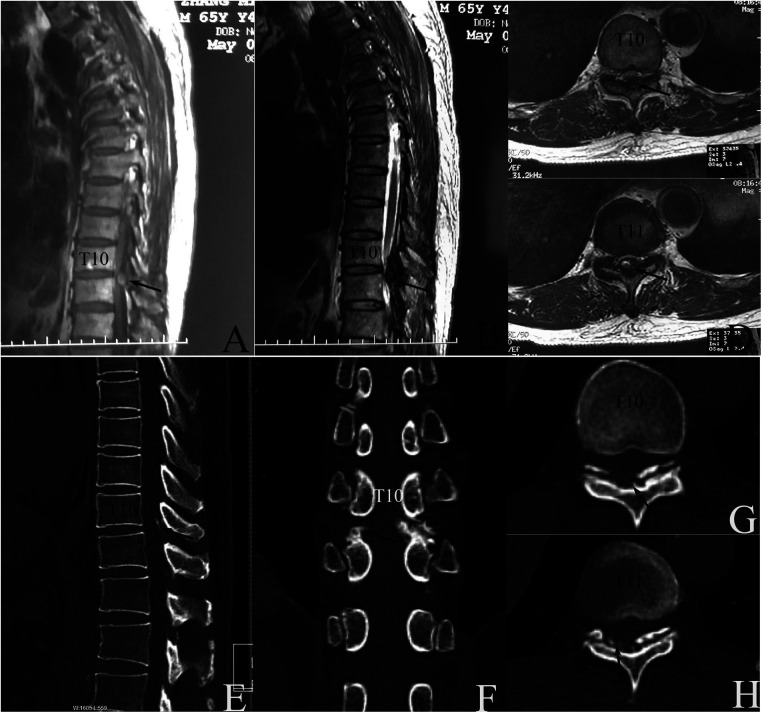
Preoperative image examinations. (A–D) Magnetic resonance (MR) taken on 2 May 2019 detected a dorsal epidural compression at T10–11 interlaminar level. (A) midsagittal T1WI image; (B) midsagittal T2WI image, and transverse T2WI image at T10 (C) and T11 (D) levels. (E–G) Computed tomography (CT) taken on May 6 revealed intraspinal canal calcification deposits posterior to the spinal cord at the T10–11 level which had no continuity with the lamina. Midsagittal (E), coronal plane (F), and transverse CT image at T10 (F) and T11 levels (G) on bone window, respectively.
